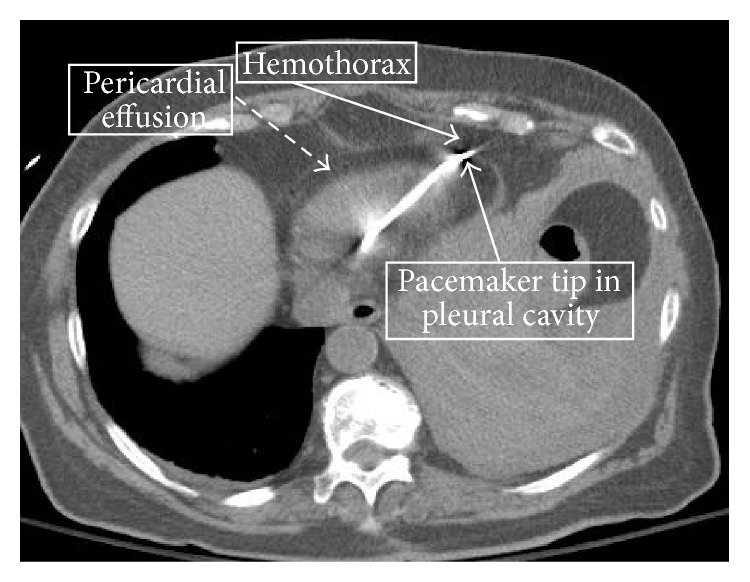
CT scan of chest showing the pacemaker wire extending to the left pleural cavity with a moderate pleural effusion and a small localized adjoining pericardial effusion.
